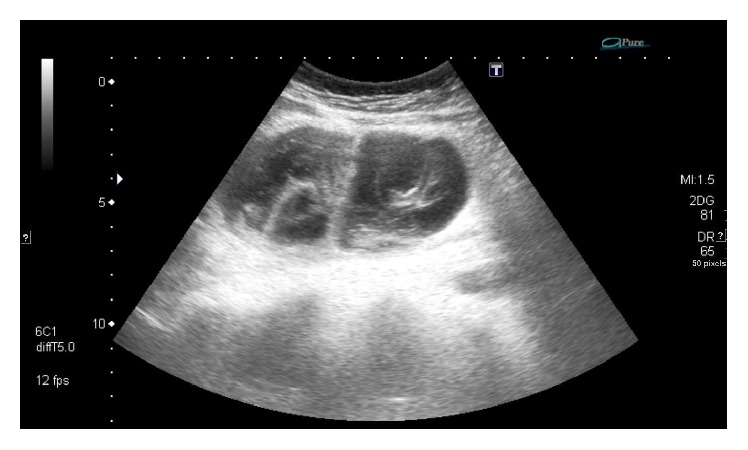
Ultrasound demonstrated a large abdominal, multilocular cystic mass. Notice the thick septations and echogenic content suspicious of a complicated cyst.
